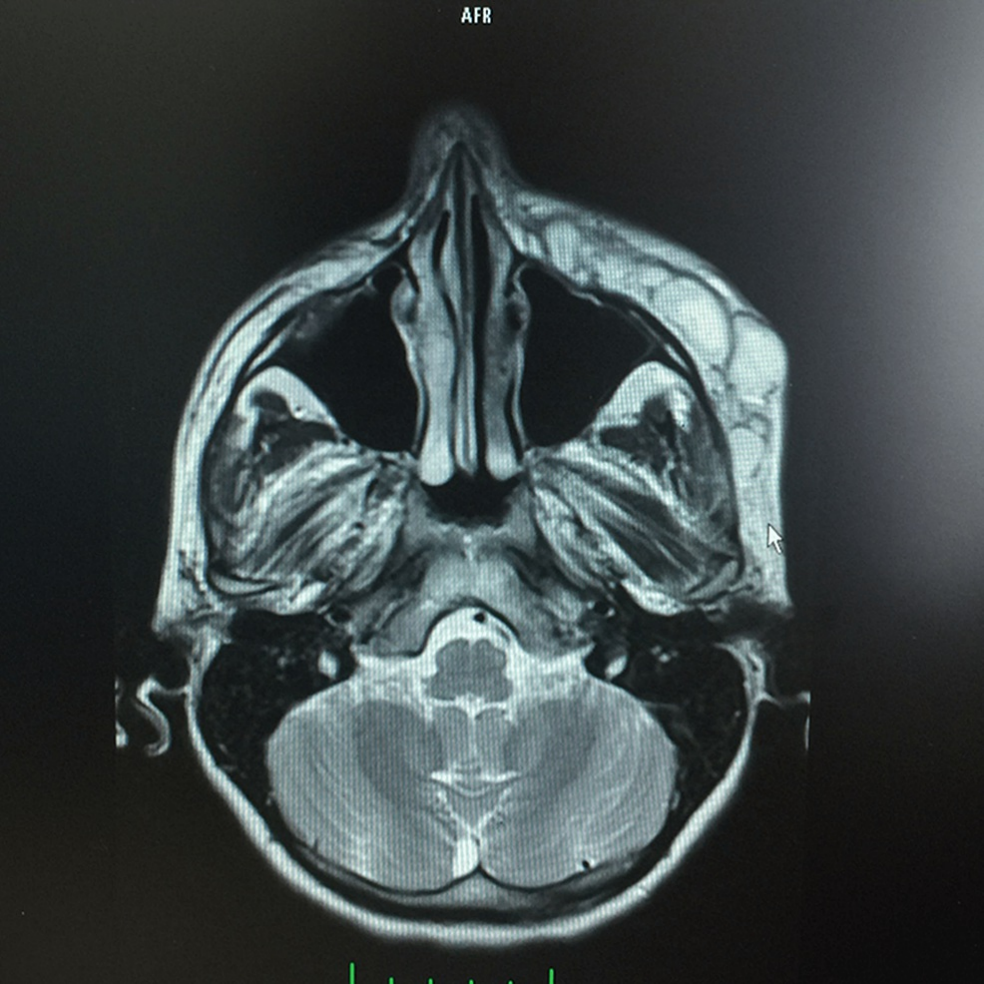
An MRI image showing multiloculated abscess on the left zygomatic arch and temporal bone.
